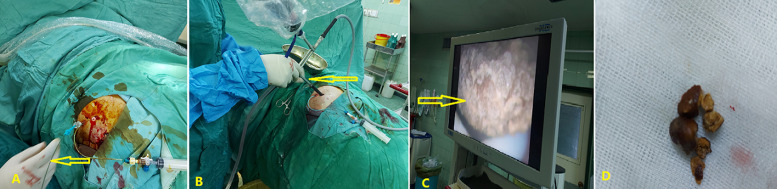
minimally invasive percutaneous nephrolithotomy with ultrasonography guidance showing: A) the J-tipped guidewire insertion into the aimed calyx (arrow); B) the rigid nephroscopy (arrow); C) the stone on the monitor (arrow); D) the removed stones
